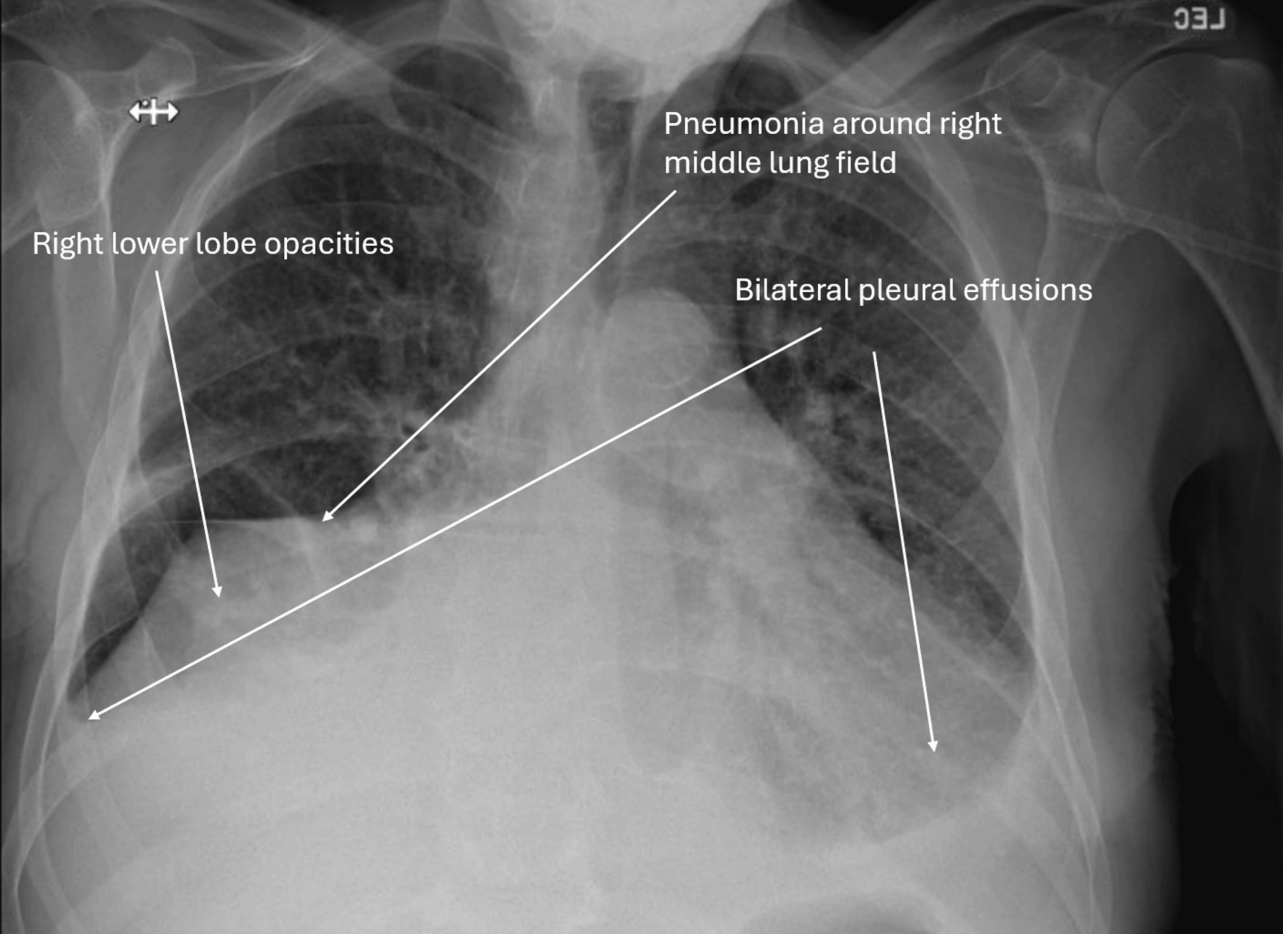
Chest X-ray on admission showing pneumonia around the right middle lung field, new opacities in the right lower lobe, mild interstitial edema, and bilateral pleural effusions.
